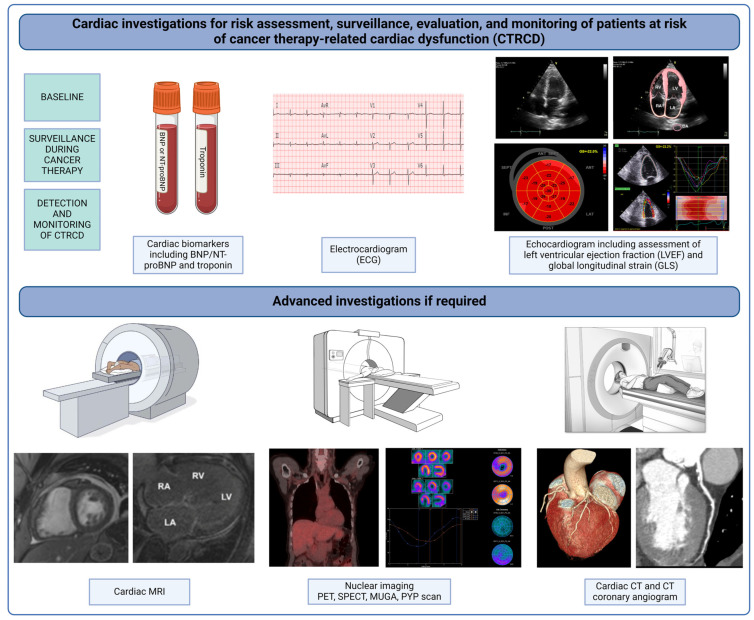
Graphical depiction of cardiac investigations involved in the baseline assessment, surveillance, and monitoring of cancer patients at risk of cancer therapy-related cardiac dysfunction. BNP, brain natriuretic peptide; NT-proBNP, N-terminal-proBNP; MRI, magnetic resonance imaging; PET, positron emission tomography; SPECT, single-photon emission computed tomography; MUGA, multigated acquisition scan; PYP, technetium-99 m pyrophosphate scintigraphy; CT, computed tomography.
